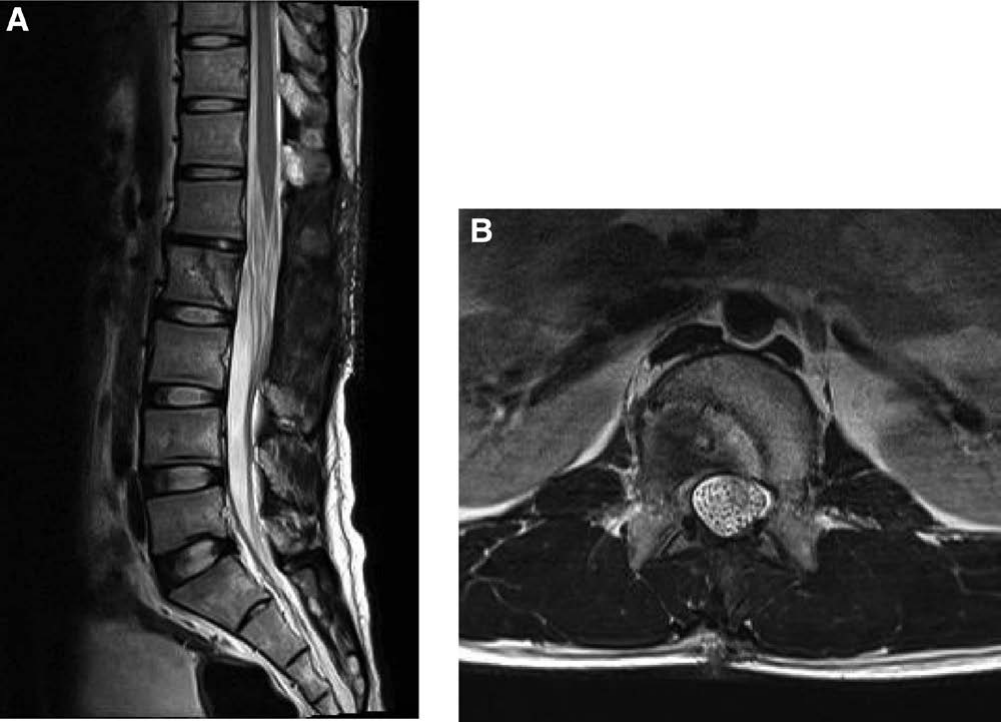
(A) Sagittal T2-weighted magnetic resonance (MR) image 3 months postoperatively, and (B) axial T2-weighted MR image 3 months postoperatively showing that epidural granulation tissues had completely disappeared, and dural tube had completely decompressed.
